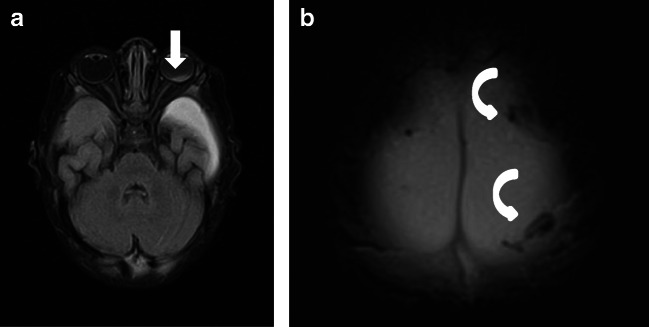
Case “AHT4”. 5 month-old. 1A: FLAIR ax, 1B:T2* ax. • Anamnesis (not available for the neuro/radiologists): Rule out hydrocephalus by macrocephaly. • MRI findings: Bilateral inhomogeneous SDH, rupture of the cortical veins (curved arrows) and retinal bleeding (straight arrow). No parenchymal injury. Extra-axial blood clots with a tubular shape in the high convexity (curved arrows) are suggestive of an acute bridging vein thrombosis and are known as “lollipop-” and “tadpole- sign” (bridging veins that terminate abruptly). • All experienced neuroradiologists correctly assumed a pAHT. Two neuroradiologists recognized the retinal hemorrhage, another neuroradiologist suspected it. • 2 out of 3 non-neuroradiologists correctly assumed an pAHT. No non-neuroradiologist recognized or suspected the retinal hemorrhage.
